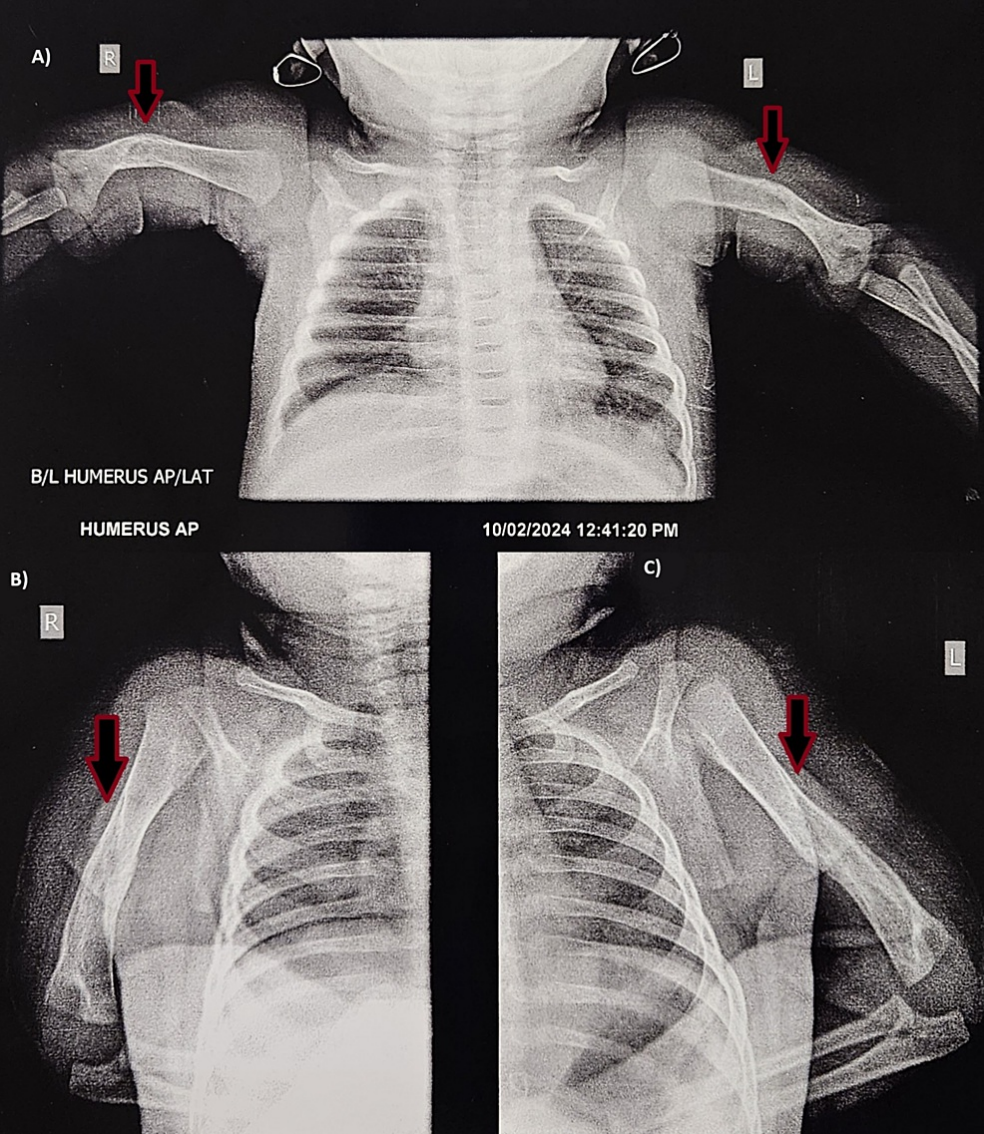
X-ray showing recovery A) Bilateral humerus anterior-posterior (AP) view; B) right humerus lateral view; C) left humerus lateral view
